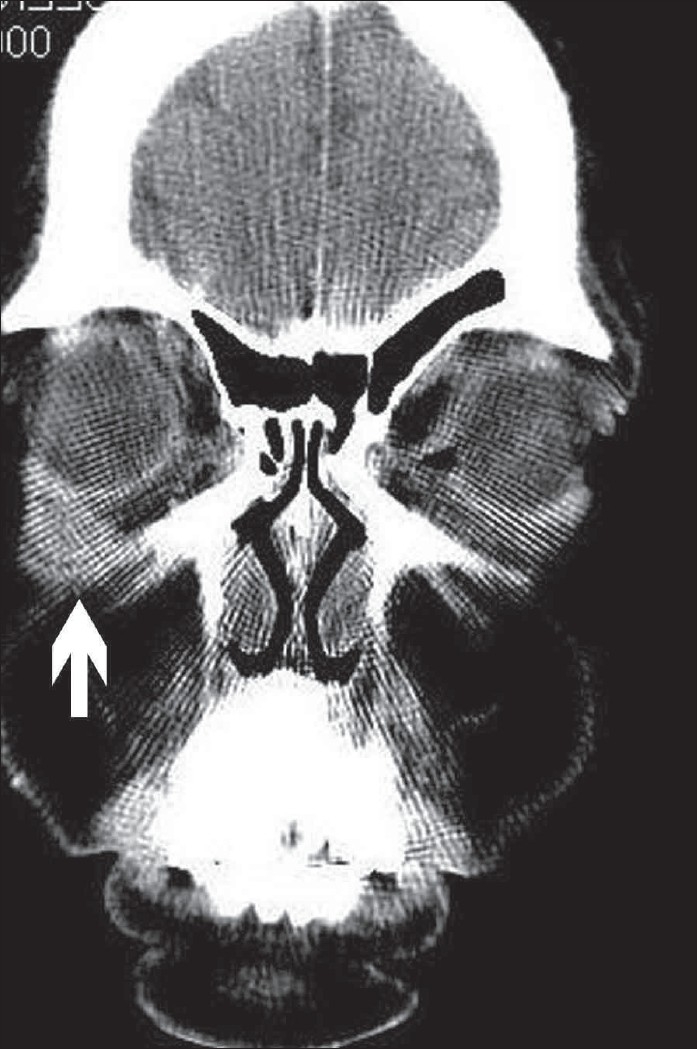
Post-contrast coronal CT image showing the same enhancing heterogeneous lesion (arrow)
